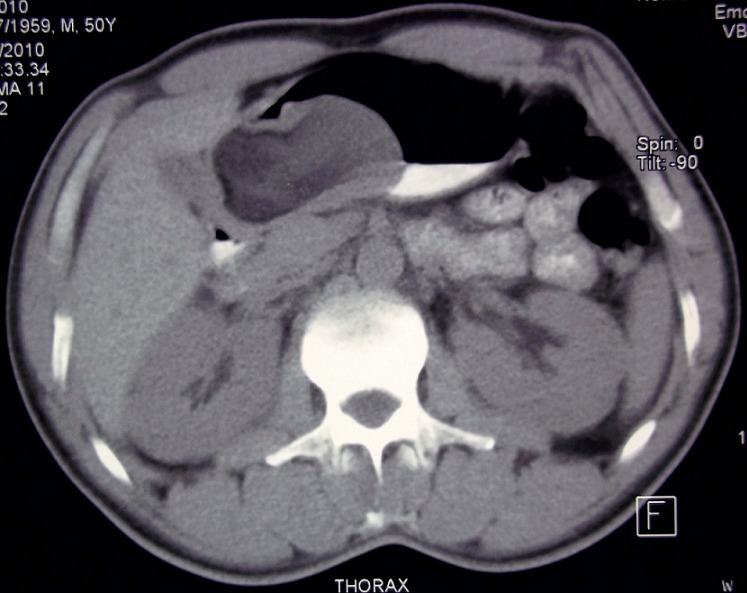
Abdominal CT scan: A large, low-attenuation, gastric mass
